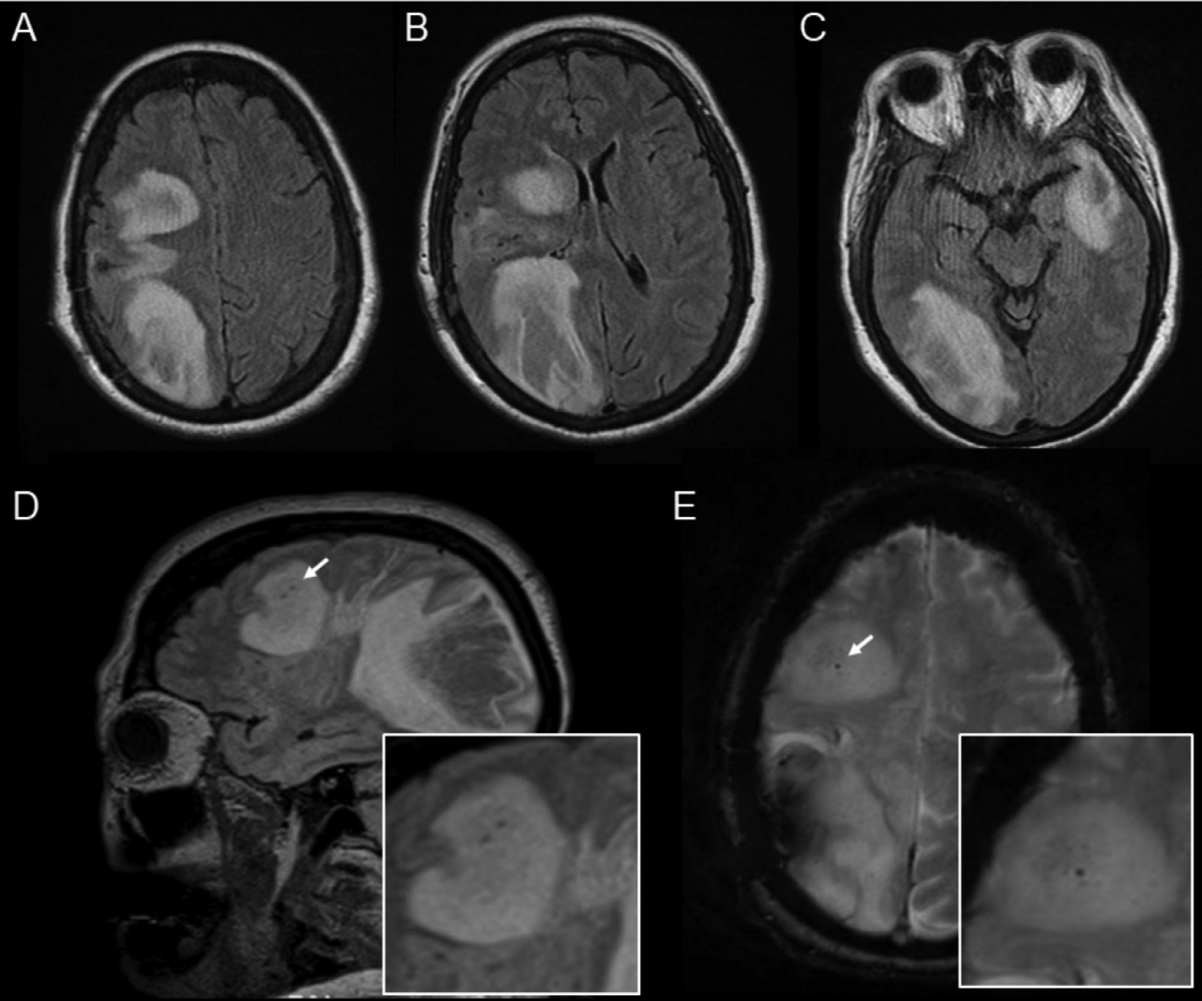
Repeat brain MRI, 11 days following the last imaging, demonstrating expansion of the tumefactive lesions despite aggressive immunotherapy. Axial (A–C) and sagittal (D) T2 fluid inversion recovery images showing interval increase in size of the of the bilateral lesions, now with more prominent edema and regional mass effect. An intralesional central vein sign can be seen on sagittal T2-FLAIR (D, arrow and image inset) and corresponding axial gradient echo imaging (E, arrow and inset).
